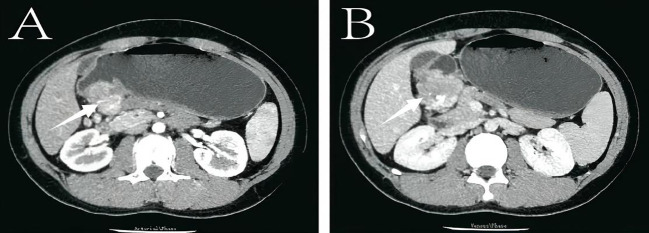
Contrast-enhanced computed tomography (CT) results. (A) Arterial phase, showing a mass-like density increase (3.8×3.6 cm) in the gastric antrum with patchy high-density calcifications, relatively well-defined margins, and significant enhancement, accompanied by enlarged surrounding lymph nodes. (B) Venous phase, displaying similar characteristics with well-defined boundaries and significant enhancement, along with enlarged lymph nodes.
